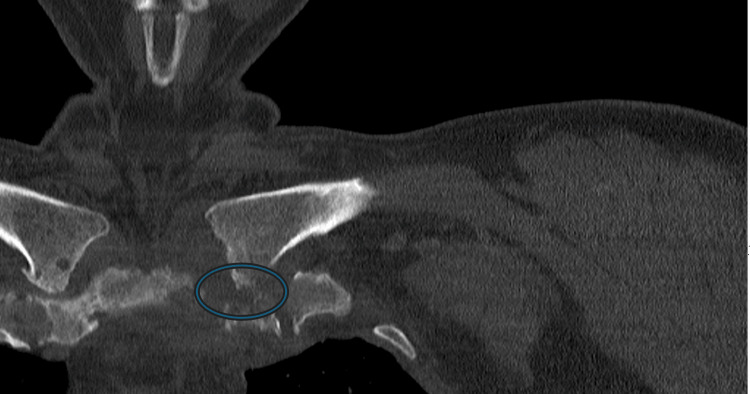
CT scan (coronal view) The coronal CT reveals asymmetric widening of the left sternoclavicular joint compared to the contralateral side. The blue circled region highlights osseous destruction at the medial clavicle and adjacent manubrial articulation. Surrounding soft tissue density changes suggest an infectious or inflammatory process, possibly with phlegmon formation.
